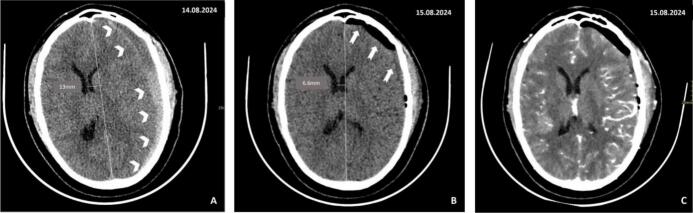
A. Baseline plain brain CT demonstrated acute subdural hemorrhage (arrowheads) with a midline shift of 13 mm (measured) B. Post burr hole and evacuation of subdural hematoma plain CT demonstrated a pocket of air at the left frontal subdural space (bolded arrows). A midline shift of 6.6 mm was also observed (measured) C. Cerebral angiography demonstrated normal findings.
